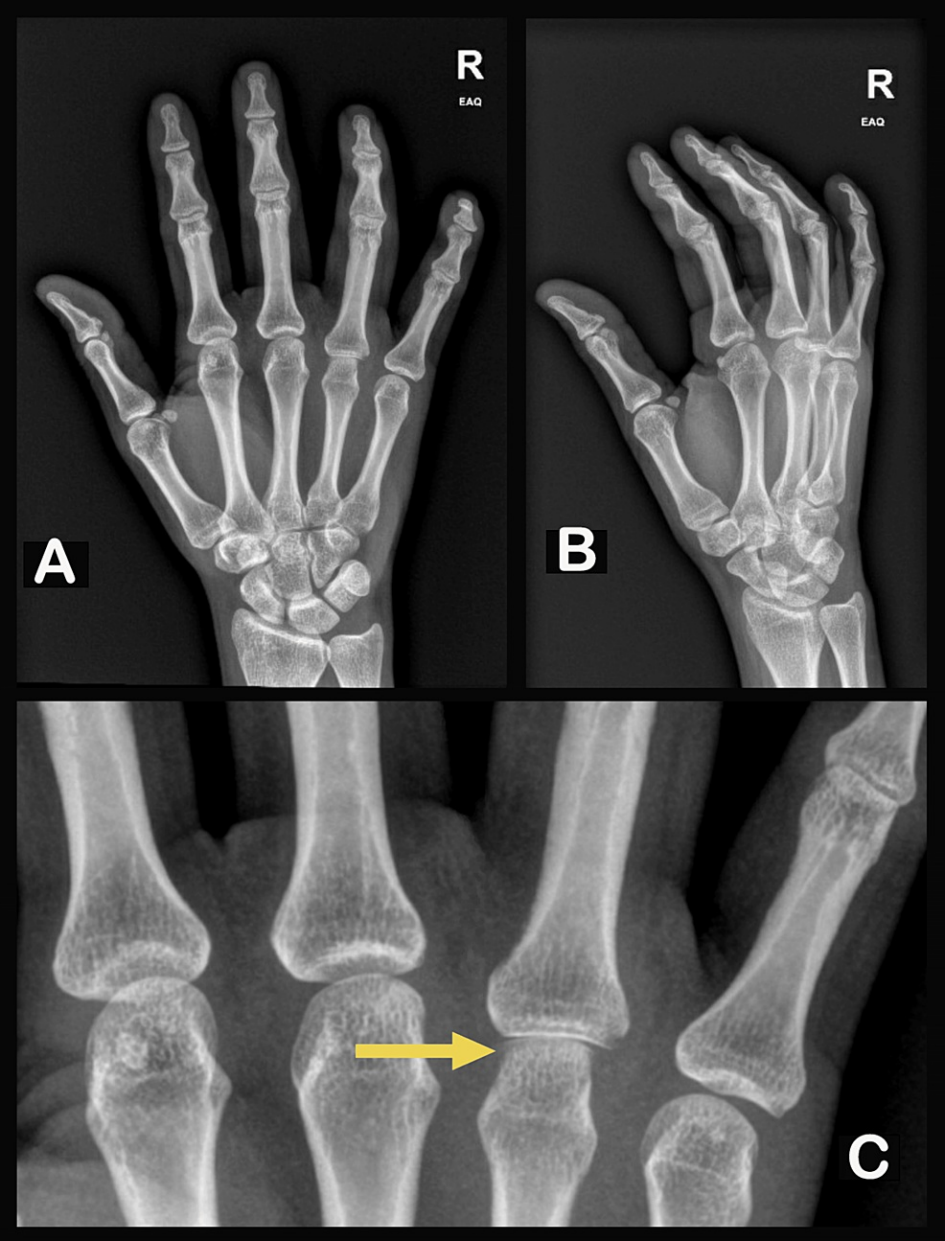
X-ray of the right hand. The bony effacement and periosteal elevation (C) at the MCP region of the fourth finger suggest osteomyelitis. MCP: Metacarpophalangeal
